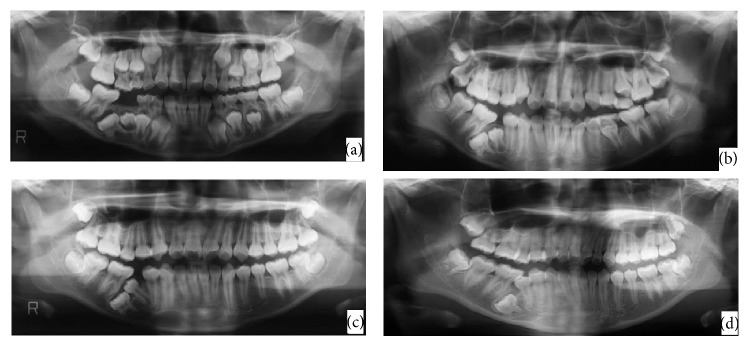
Panoramic radiographs showing growth changes of the dental germ: case  2. The formation stages of the impacted permanent tooth were approximately (a) Ri at age of 9 years and 2 months, (b) R1/2 at age of 11 years and 0 months, and (c) R3/4 at age of 12 years and 6 months. (d) At age of 16 years and 7 months, most of the tooth crown of the second permanent premolar had erupted, but the second primary molar remained impacted.
